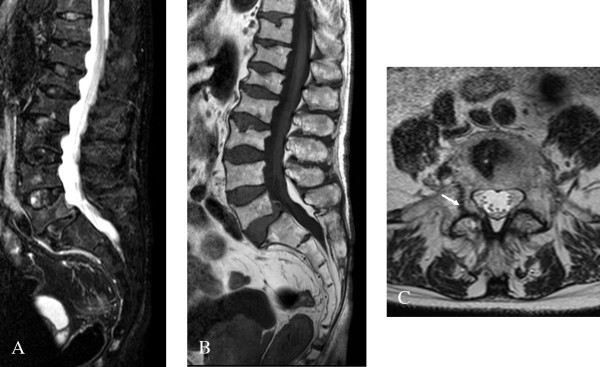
Bone magnetic resonance imaging in a Fabry patient with severe osteoporosis: A (STIR, sagittal view) and B (T1, sagital median): several vertebral body fractures are seen, without signal anomaly in T1 or T2 in favor of ancient fractures. A mild spondylolisthesis of L5 on S1 can be observed. C (T2, axial view): fracture of the right pedicula of L5 (arrow) in a 72-year-old patient with severe osteoporosis. Courtesy: Dr Robert CARLIER, CHU Raymond Poincaré, Garches, France.
